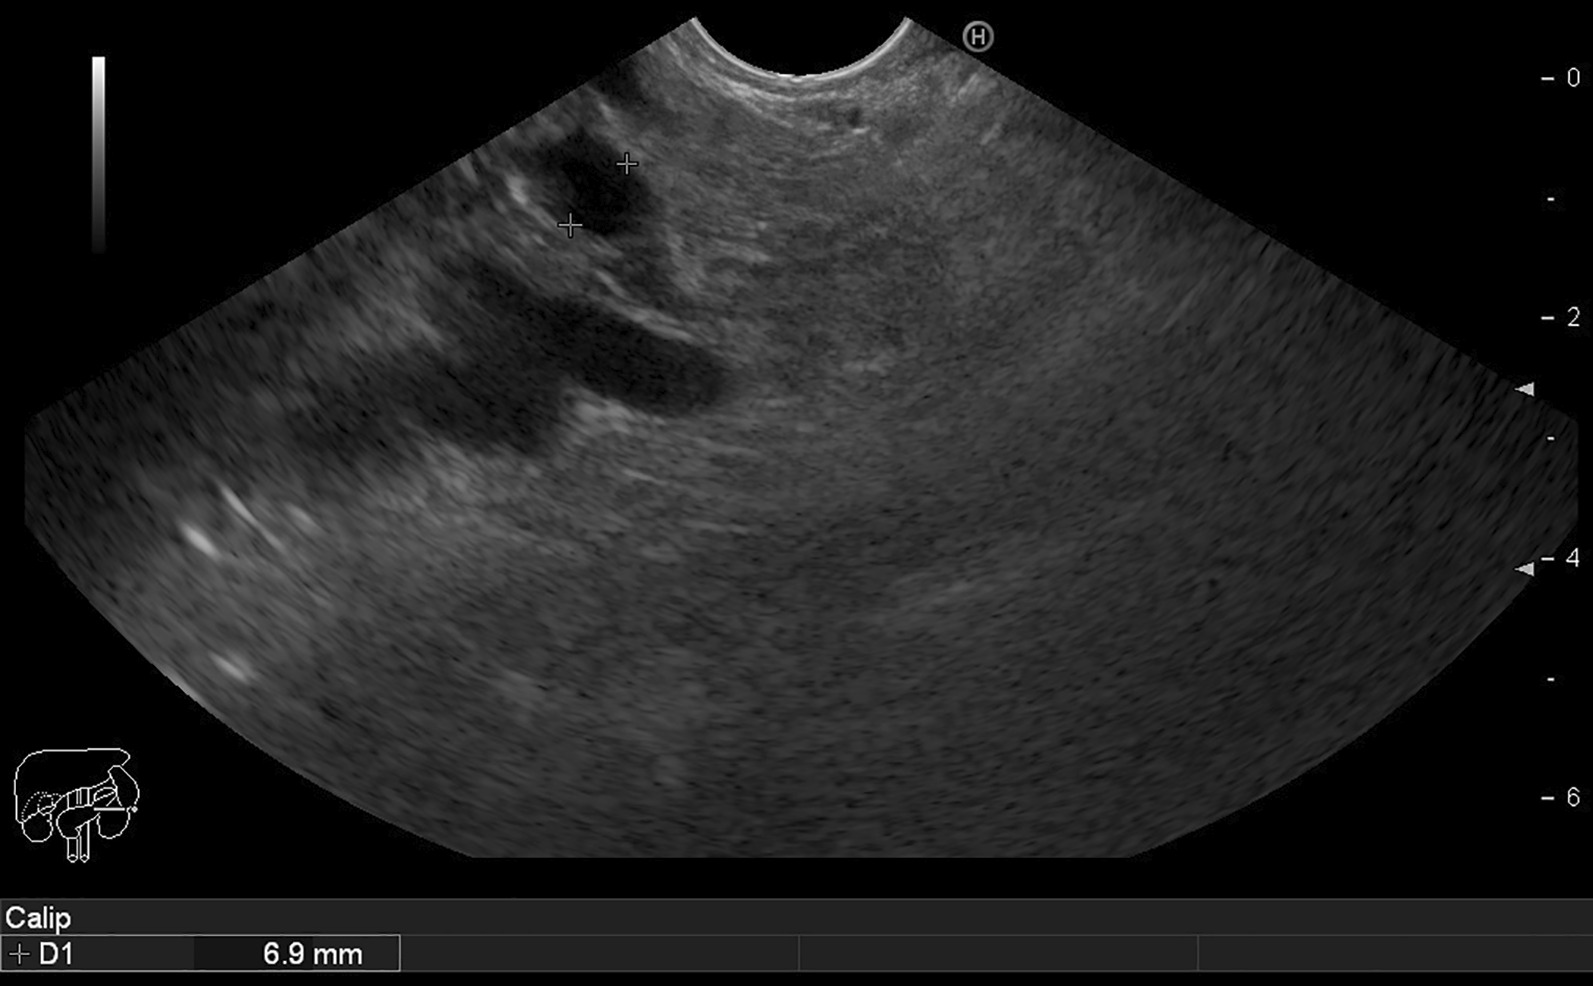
Endoscopic ultrasound showing a dilated pancreatic duct
